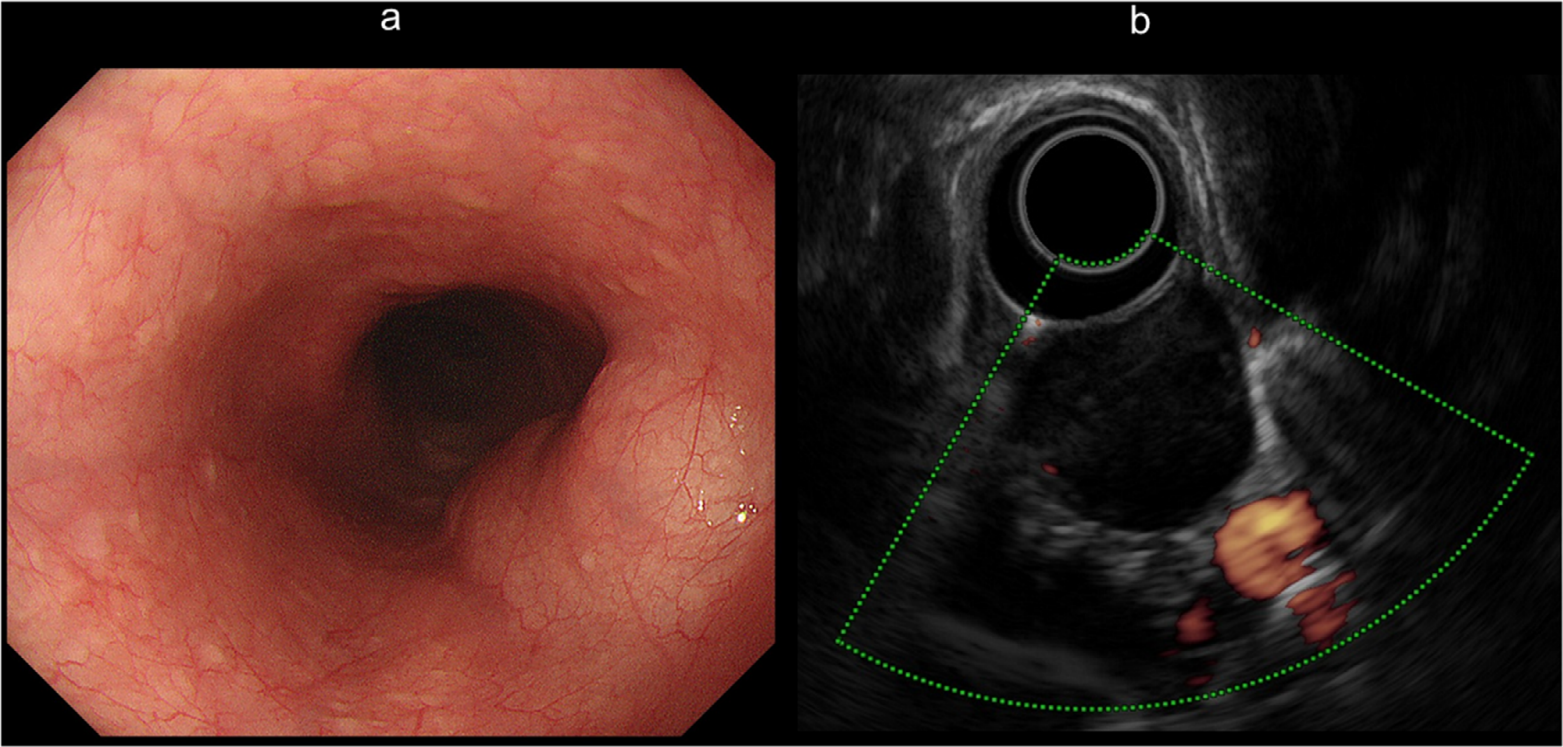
(a) Screening esophagogastroduodenoscopy showed a submucosal tumor located at 5 o'clock in upper thoracic esophagus. (b) Endoscopic ultrasound showed a uniform low‐echoic lesion with space between the tumor and azygos vein
